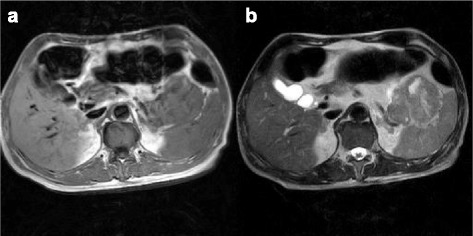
Abdominal magnetic resonance imaging (MRI) 14 days after contrast-enhanced CT. a T1 weighted MRI, b T2 weighted MRI image, showing a heterogeneous mass, 100 mm in diameter, at the tail of the pancreas
